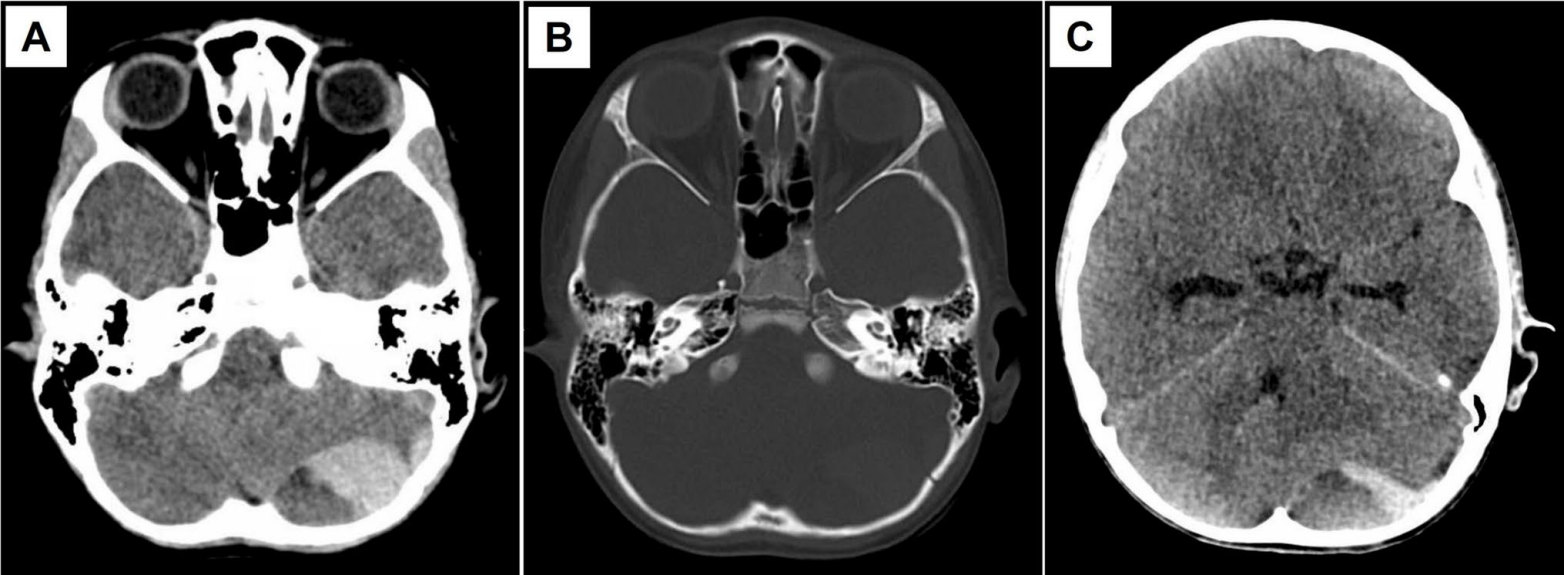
Case example of a pediatric patient with PFEDH from our series. Representative pre-operative CT brain images in axial direction depicting: (A) a left-sided, biconvex hematoma with mixed density causing local mass effect on the underlying parenchyma; (B) the corresponding bone window of (A) showing an occipital bone fracture adjacent to the PFEDH; (C) another image slice that shows radiological evidence of obstructive hydrocephalus, fourth ventricular distortion and effacement of the peri-mesencephalic cisterns. (Abbreviations: PFEDH = posterior fossa extradural hematoma; CT = computed tomography.)
